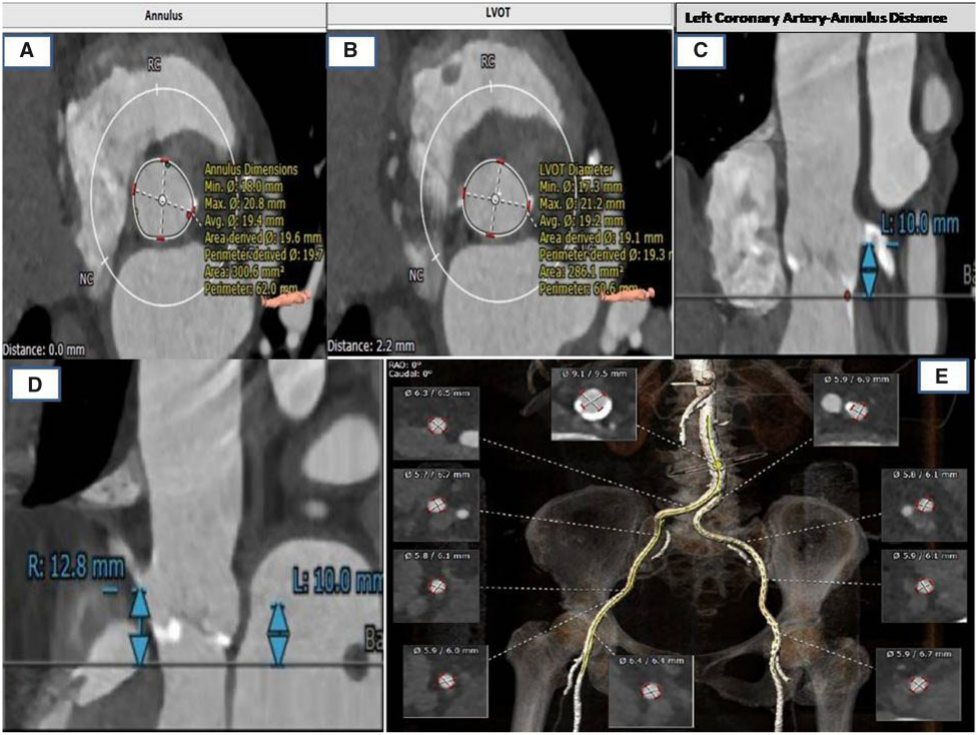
Computed tomography images of aortic annulus and left ventricular outflow tract (*A, B*). Left coronary ostial-annular distance (*C*). Right coronary ostial-annular distance (*D*). Computed tomography re-construction images of access vessels diameter and anatomy (*E*).
